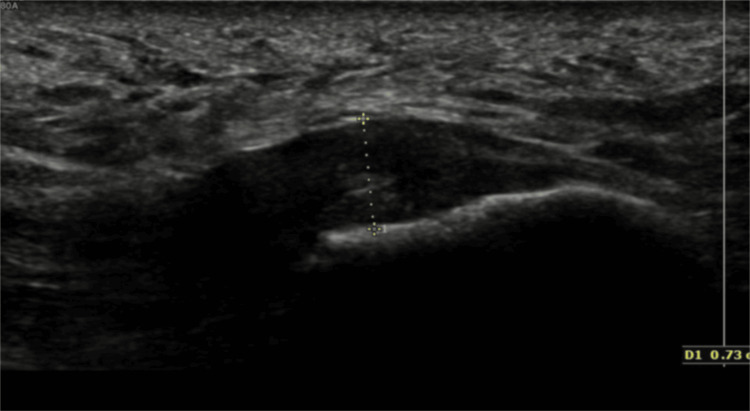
Representative pre-treatment US image showing thickening of the plantar fascia (baseline) - case 3 US: ultrasound
